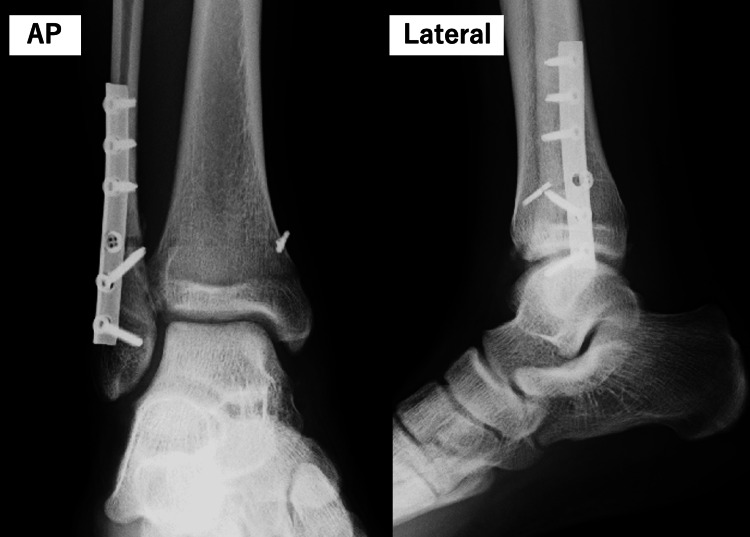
Postoperative X-rays of the right ankle Postoperative anteroposterior and lateral X-ray views of the right ankle following ORIF. The fixation was performed using a 1/3 tubular plate and Ziptight system. ORIF, open reduction and internal fixation
